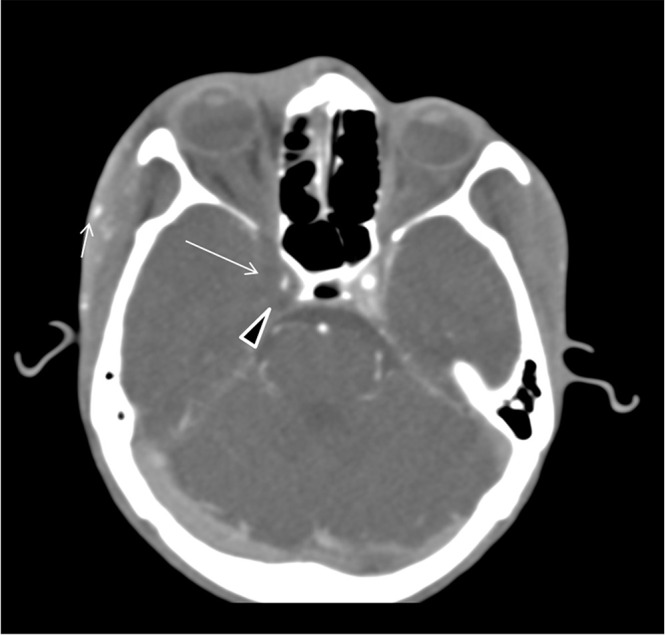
Axial post contrast CT angiography of the brain shows symmetrical non-enhancing right cavernous sinus (arrowhead), reduced caliber of the right internal carotid artery cavernous segment (arrow) swelling of the right temporal lobe and swelling and enhancement of the subcutaneous right temporal region as well as the orbital septum (short arrow).
